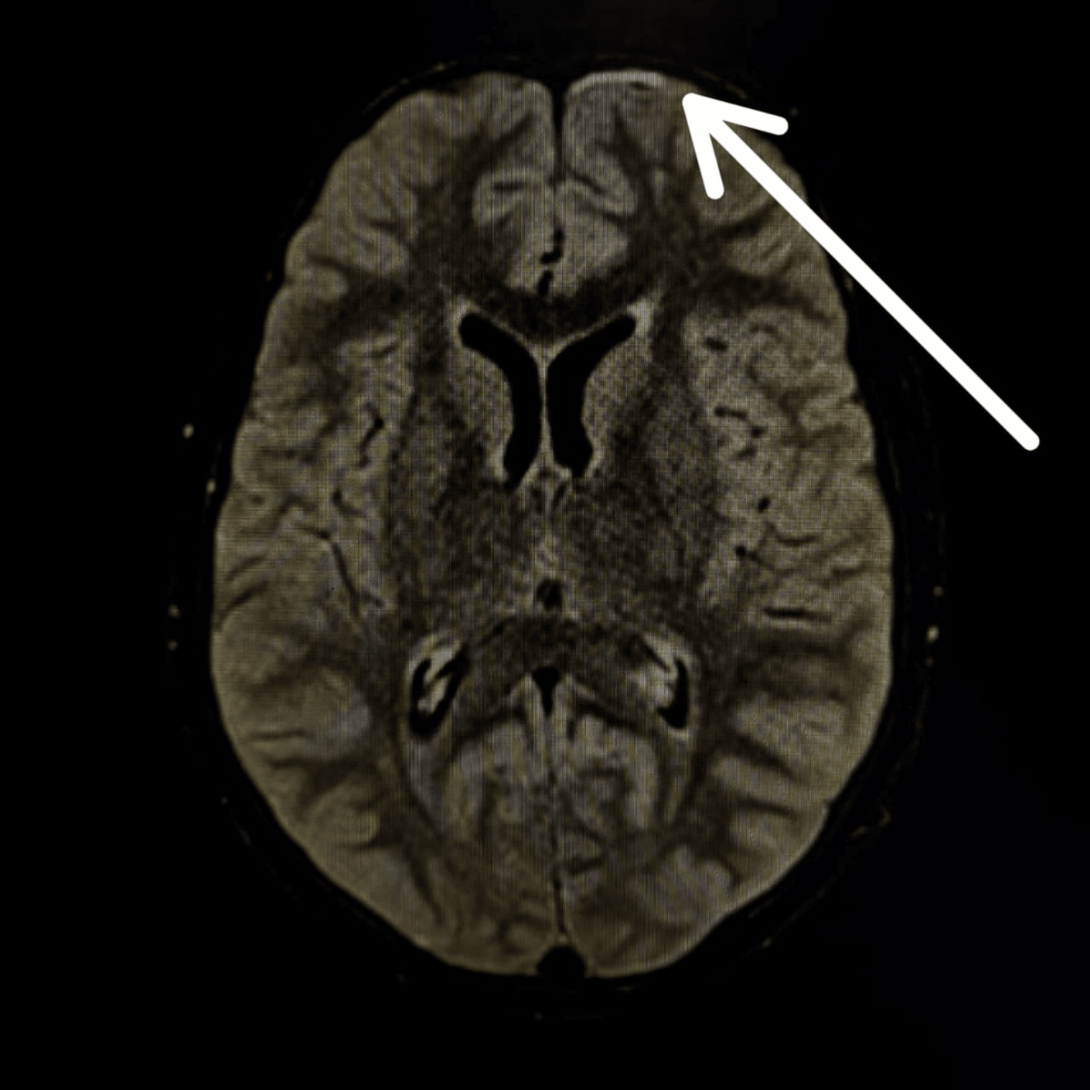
Leptomeningeal enhancement on FLAIR MRI FLAIR MRI: fluid attenuated inversion recovery magnetic resonance imaging
